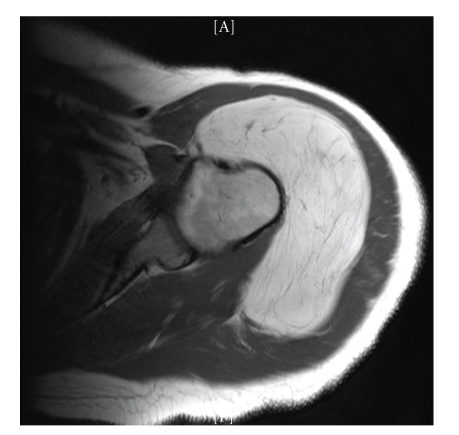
MRI Axial T1 Weighted image of the shoulder showing a large lipomatous tumour in the sub-deltoid region.
